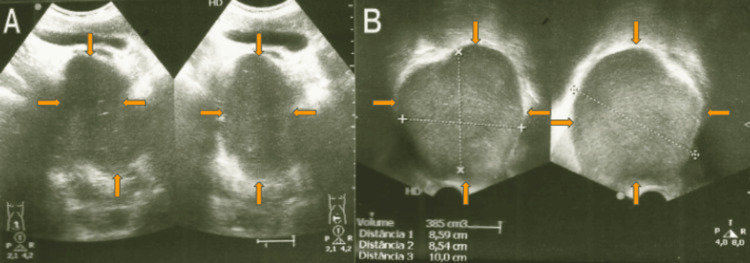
Prostate ultrasound performed via (A) suprapubic and (B) transrectal approaches The prostate demonstrates markedly increased dimensions with bosselated contours. The estimated prostatic weight is approximately 385 g. There is no clear differentiation between the central and peripheral prostatic zones.
